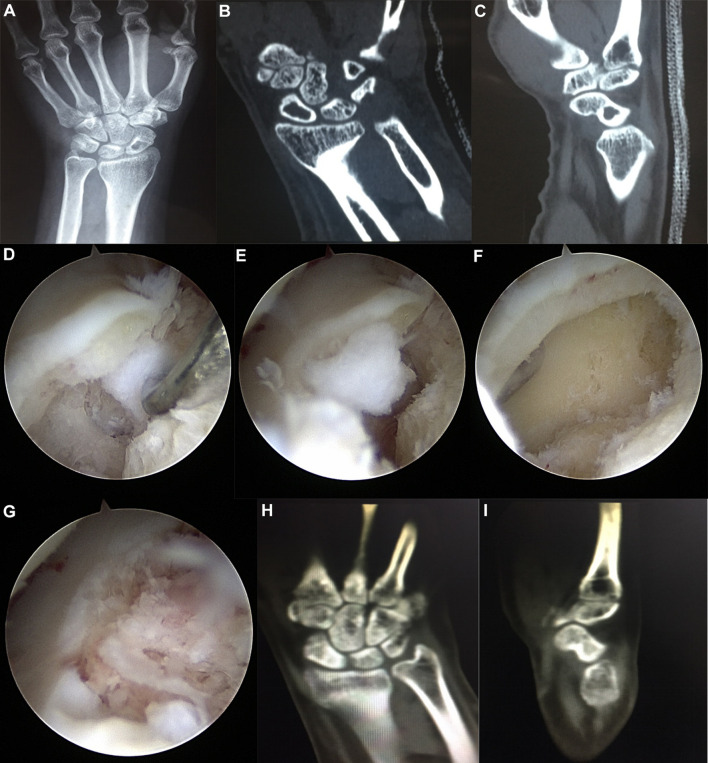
A typical case. (A) pre-operative X-ray; (B) pre-operative coronal CT; (C) pre-operative sagittal CT; (D) Intraoperative fenestration of scaphoid bone showed cyst; (E) Scaphoid cyst scraped out during operation; (F) Navicular cavity after curettage of cyst during operation; (G) Cavity bone grafting after curettage of cyst during operation; (H) The coronal CT scan showed bone healing in the scaphoid bone graft area after 3 months of follow-up; (I) The sagittal CT scan showed bone healing in the scaphoid bone graft area after 3 months of follow-up.
